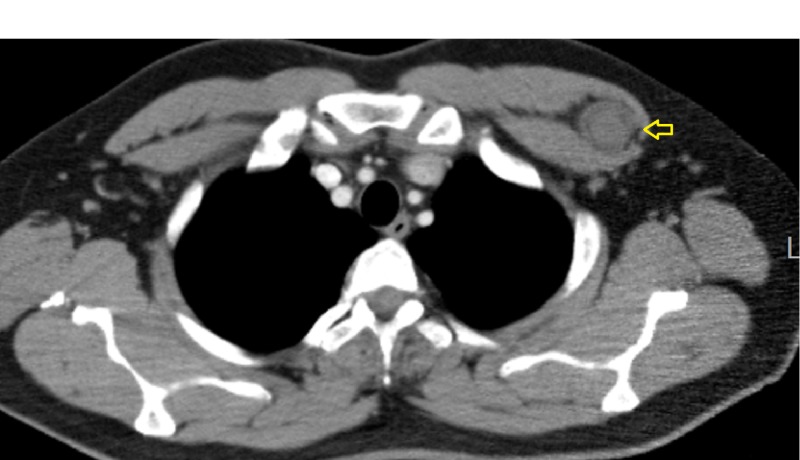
Axial CT of chest (with contrast) Well-defined, rounded, soft tissue density mass (arrow) between the left pectoralis major and minor muscles. CT: computed tomography
